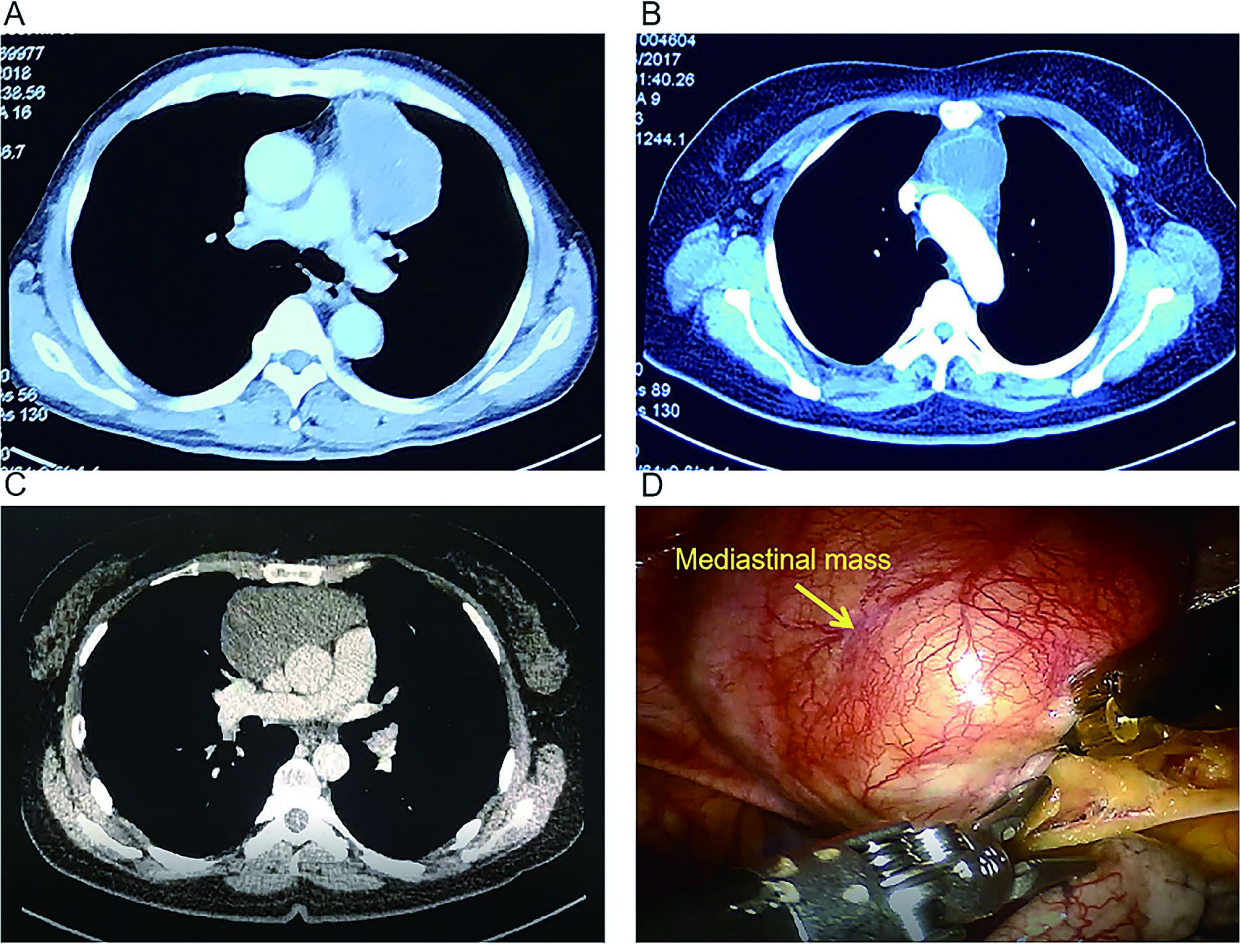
(A, B) Representative computed tomography images of 2 patients with anterior mediastinal lesion. (C, D) A 31-year-old woman underwent robot-assisted thoracic surgery on December 16, 2019
